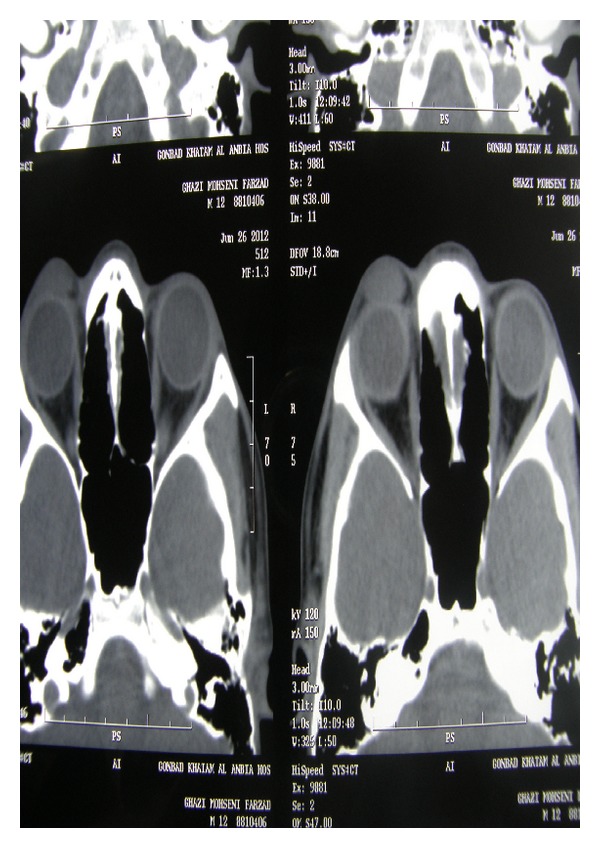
Axial and coronal view of orbital CT scan that showed well-circumscribed, uniform preseptal mass without orbital extension.
